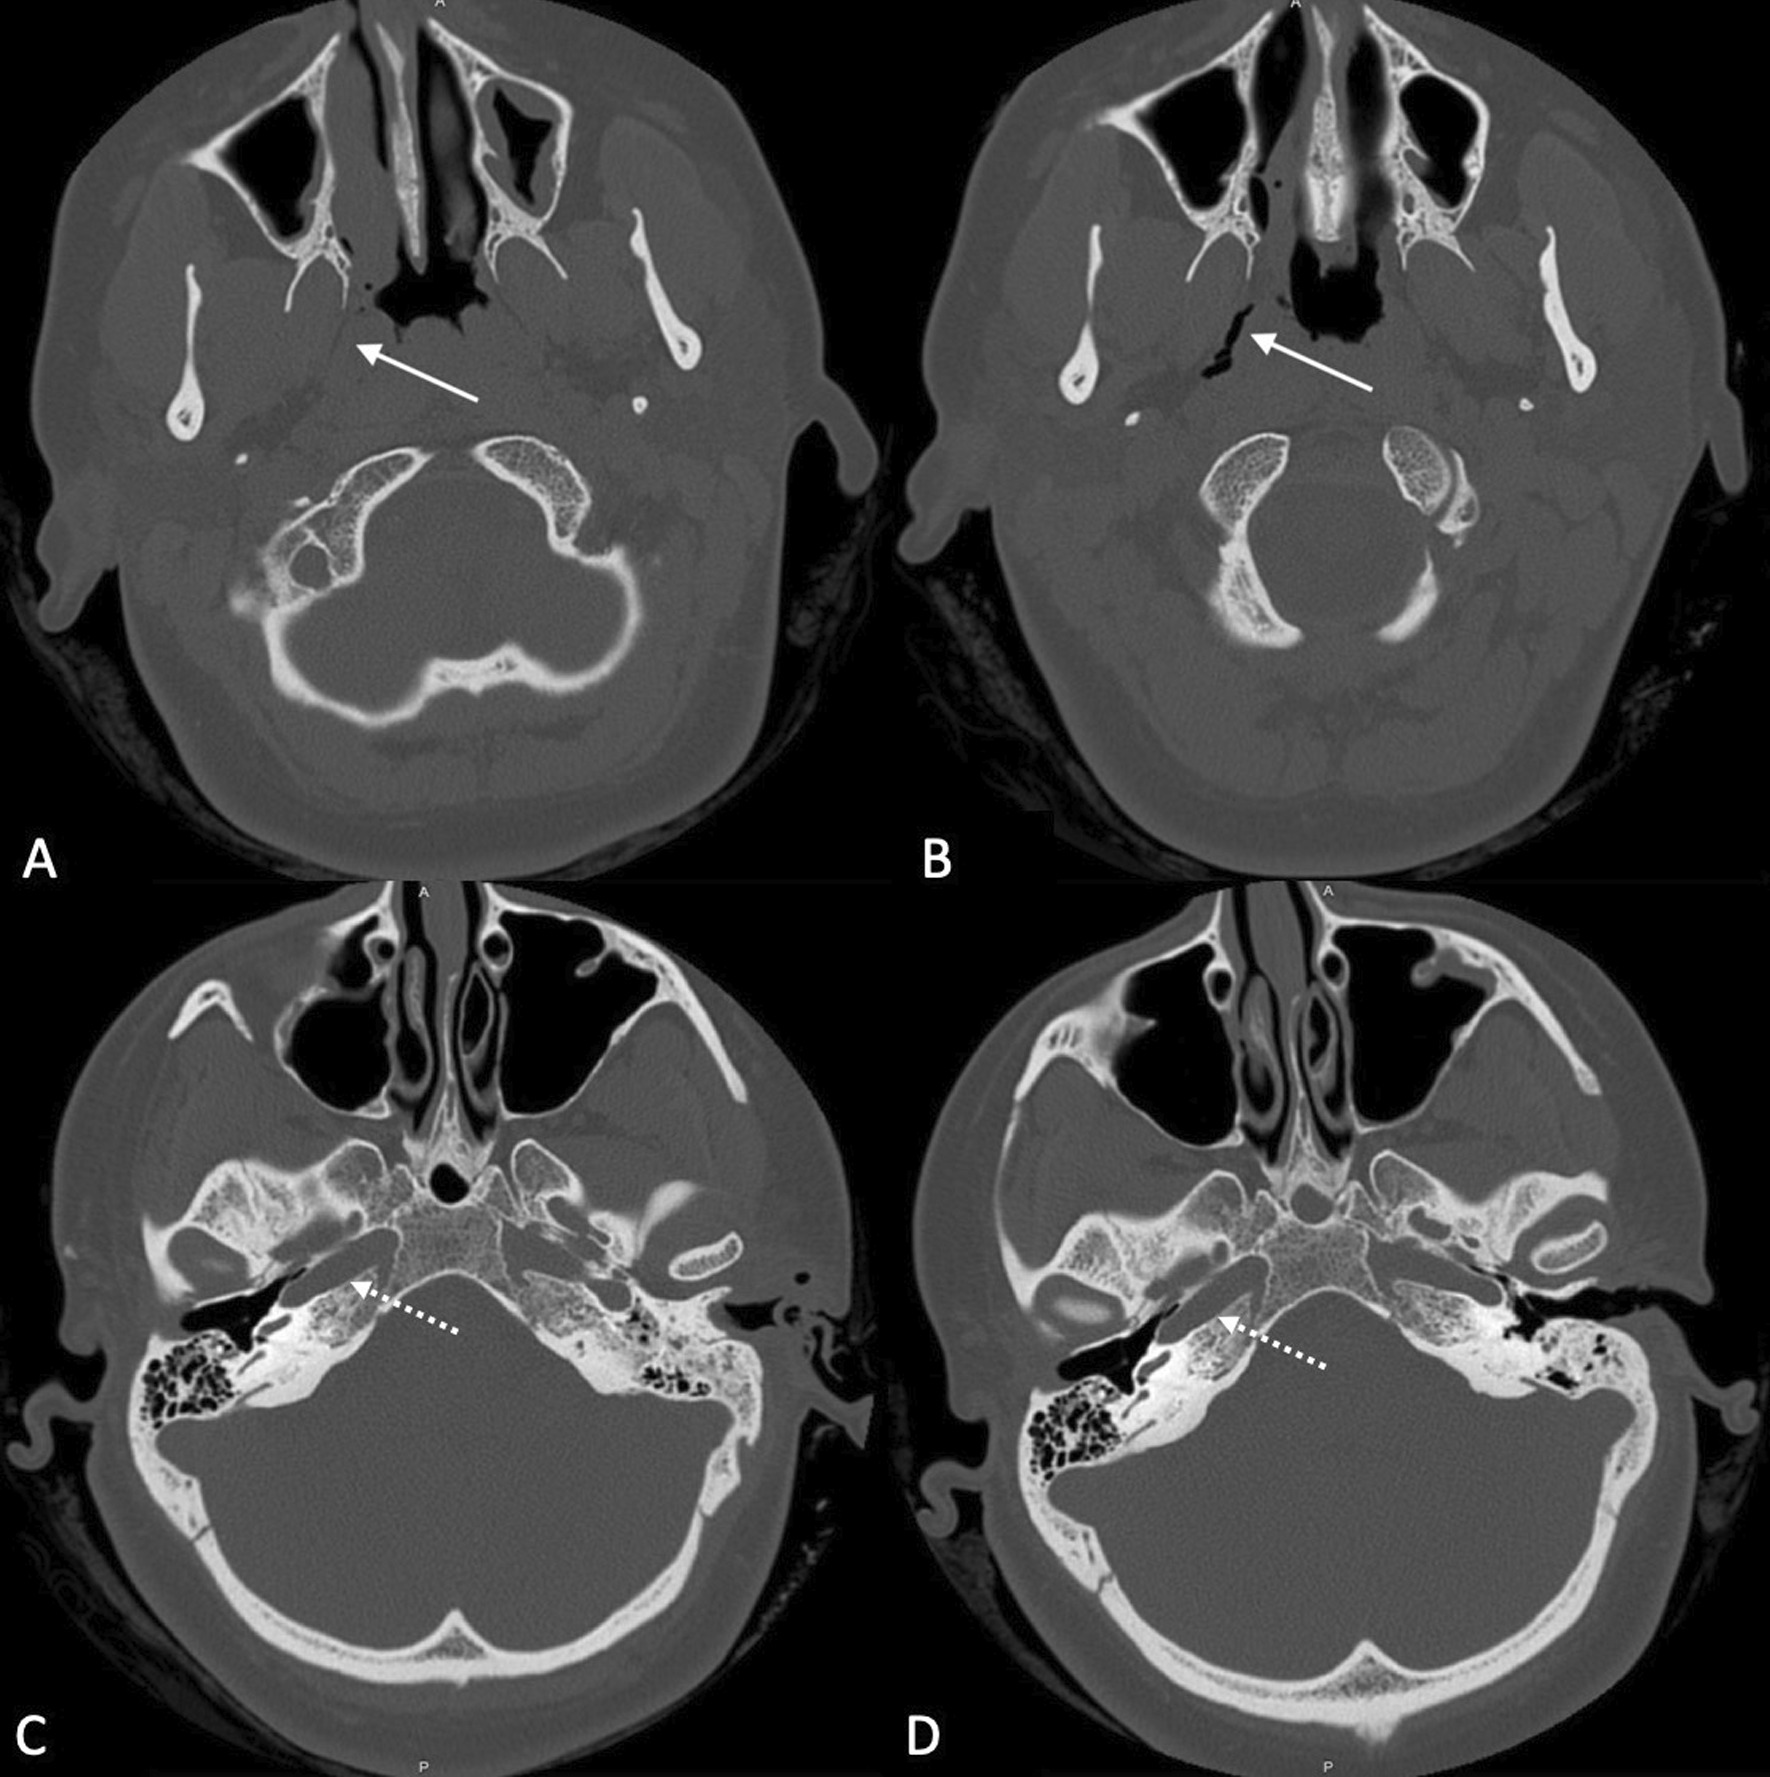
Comparison of pre- and postoperative CT (axial view). A preoperative CT, solid arrow points to the eustachian tube. B postoperative CT, solid arrow points to the dilated eustachian tube. C preoperative CT, dash arrow points to carotid canal before dilation. D postoperative CT, dash arrow points to intact carotid canal after dilation of the eustachian tube—no dehiscence can be noted
